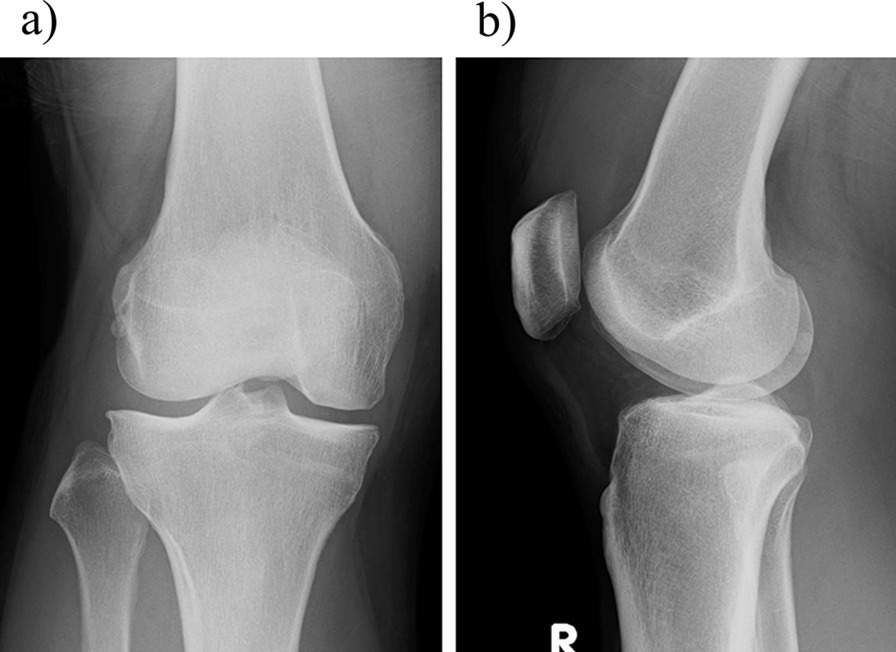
The AP and lateral radiograph of the right knee in April 2018. a AP radiograph, b lateral radiograph
